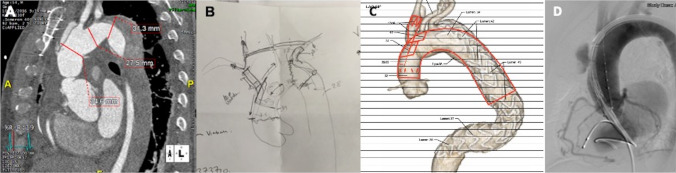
A careful assessment and planning before the procedure is the key element for a successful endovascular treatment for proximal dissection. A shows an extensive measurement based on gated computed tomography (CT) images; B shows the exploration of potential procedure in a sketch; C shows computational modeling to mimic the devices and outcome; D shows a real-world case accomplished after intensive multidisciplinary preparation
